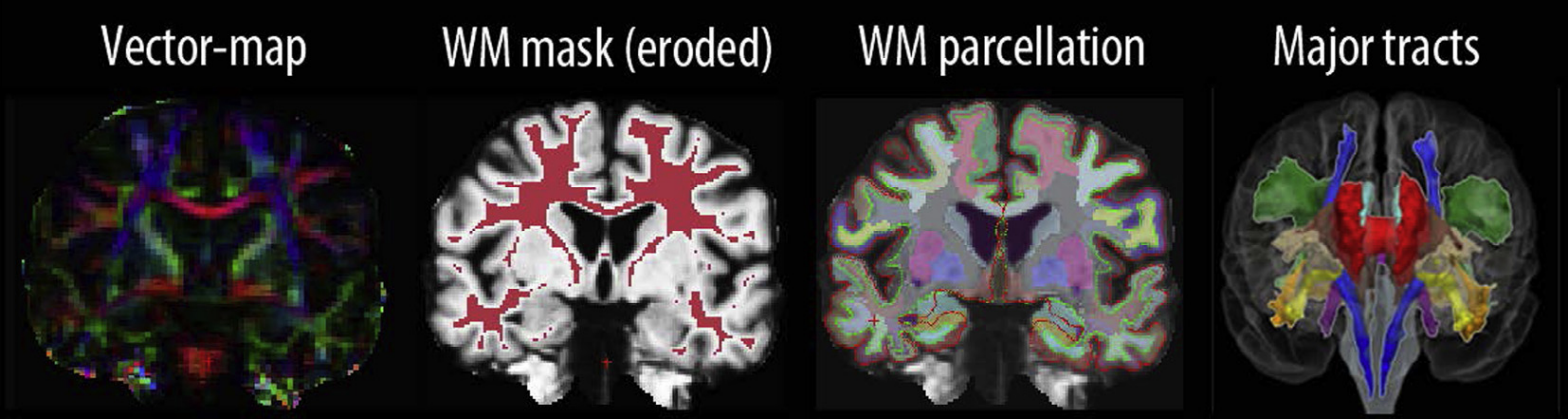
Multiple examples of ways of measuring and reconstructing white matter properties based on MRI scans.
